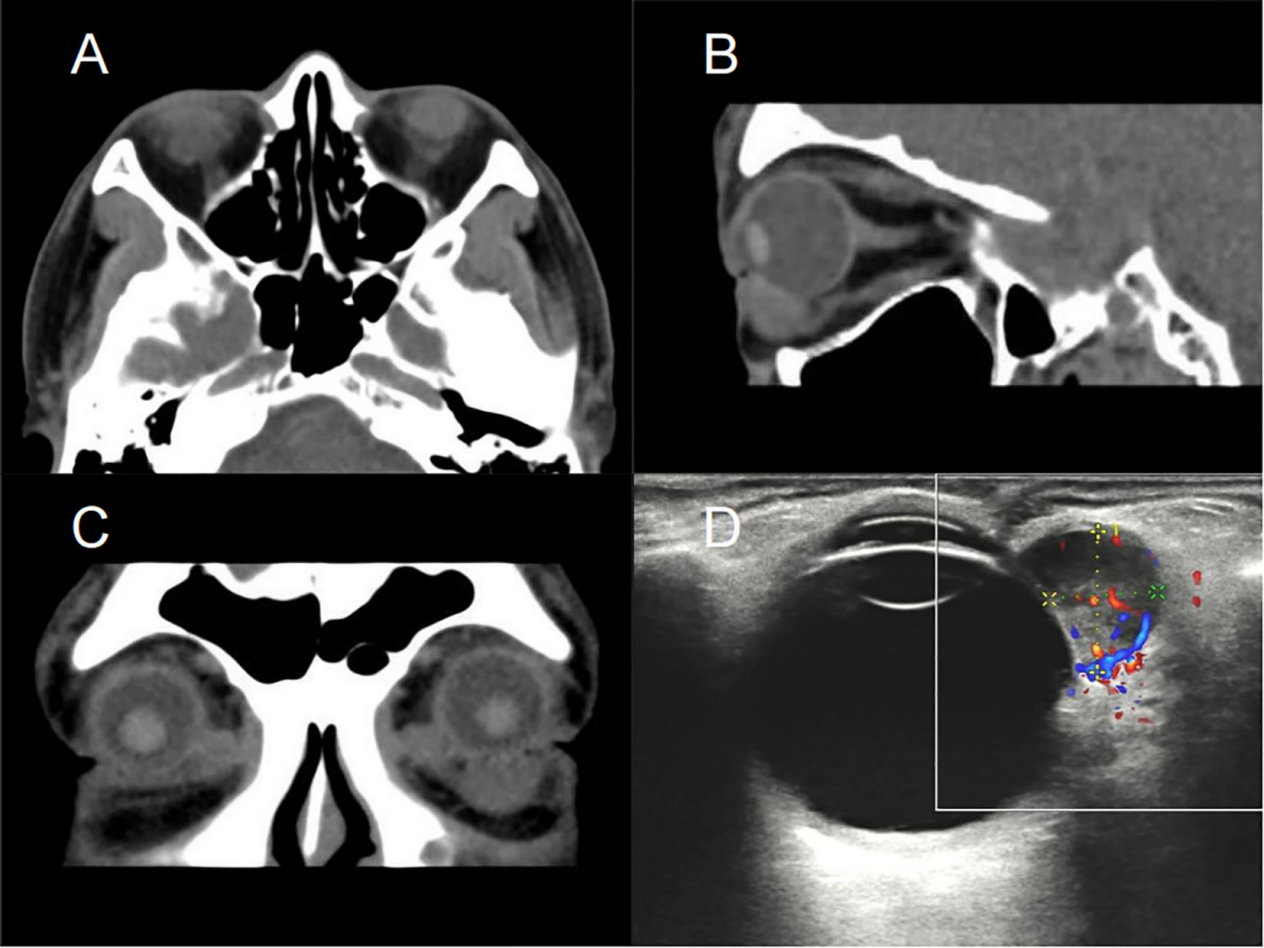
CT scans and ultrasound of the patient’s orbit. Axial (A), sagittal (B), and coronal (C) CT images reveal a well-defined, ovoid soft tissue mass located beneath the left globe, with no evidence of invasion into the eye globe or adjacent orbital bones. (D) Ultrasound imaging reveals a hypoechoic mass adjacent to the lateral aspect of the left eyeball, measuring approximately 1.50×0.90×0.75 cm. CDFI displayed abundant blood flow signals within the mass, indicating a well-vascularized lesion. CT, Computed Tomography; CDFI, Color Doppler Flow Imaging.
